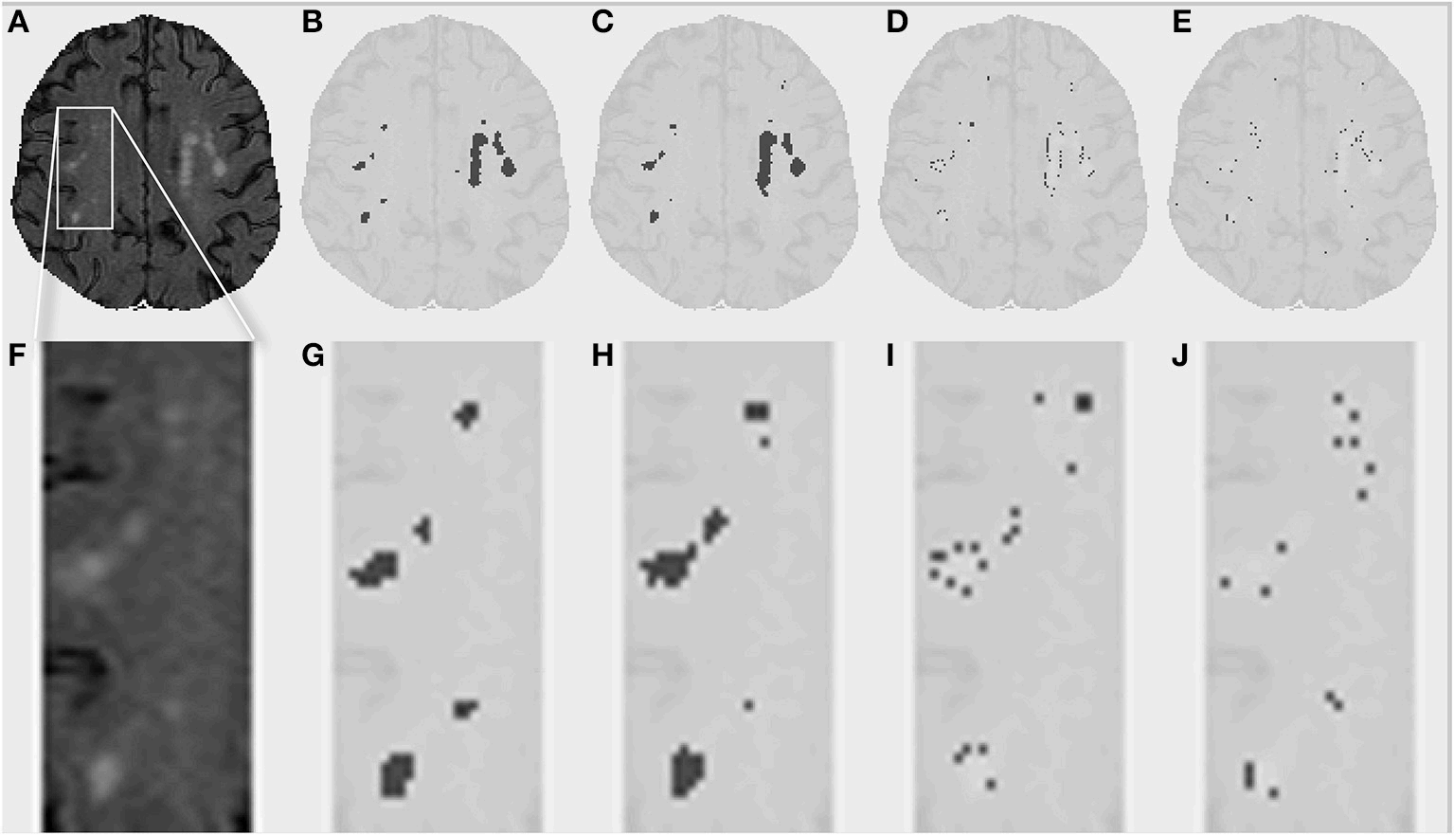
White matter lesions (WML) in the centrum semiovale. (A) FLAIR image for a given subject. (B) Conventionally estimated WML. (C–E) Estimated WML using the proposed segmentation algorithm, for full, intermediate, and small proportion of lesion. (F–J) Similar images for the zoomed portion depicted by the white box in (A).
